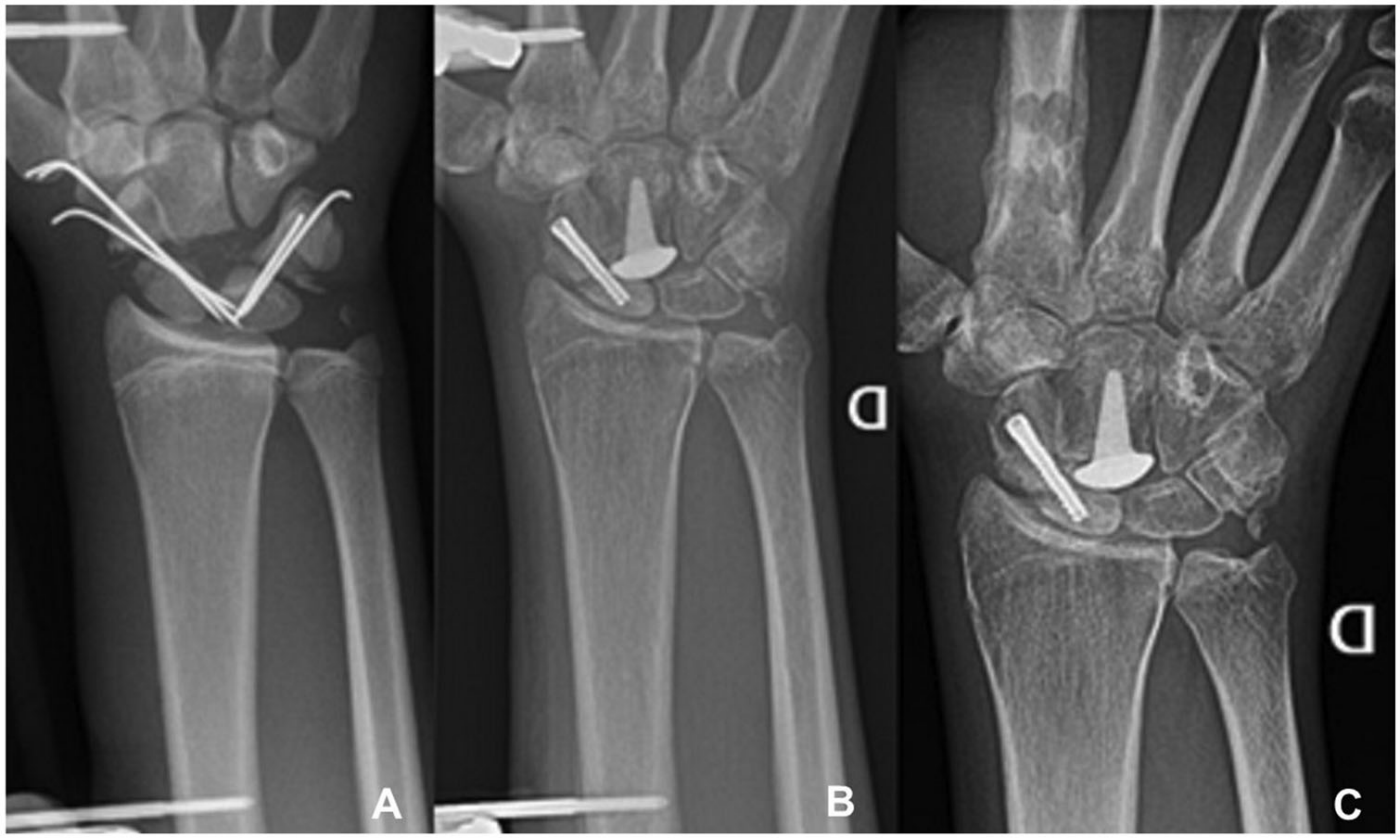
(A) X-ray after the first surgery. Note the empty space at the place of the proximal pole of the capitate. (B) X-ray after the second surgery, the K-wires were replaced by the RCPI prosthesis and a screw was inserted in the scaphoid. (C) At 21 months’ follow-up (all case 1).
